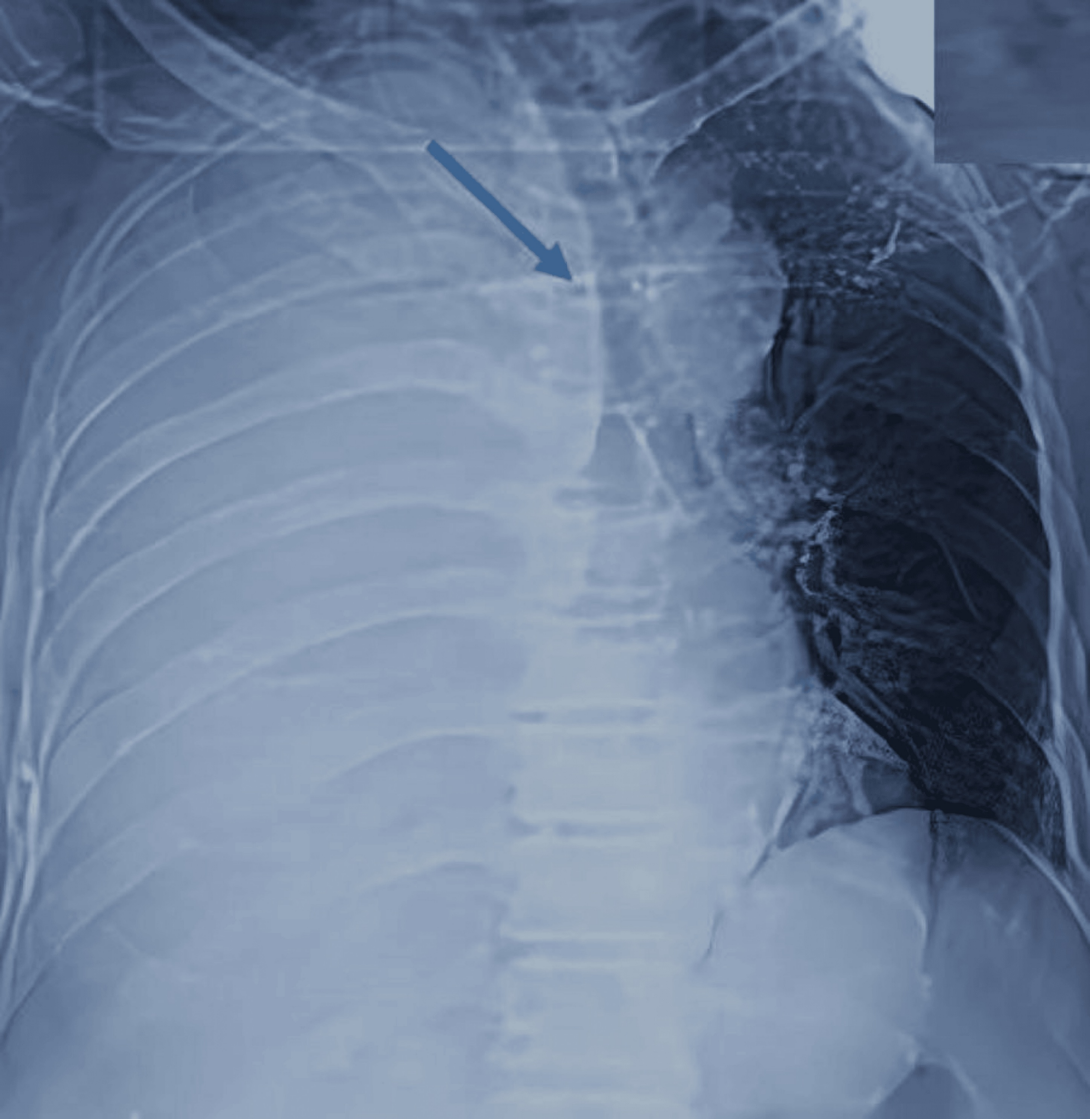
Chest X-ray in the AP view showing a white right lung with displacement of the mediastinal structures (trachea) toward the contralateral side, consistent with large-volume pleurisy. AP, anteroposterior
